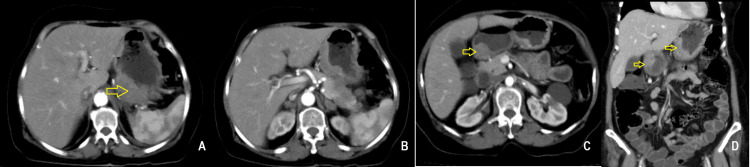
Contrast-enhanced computed tomography images in axial sections showing asymmetrical, heterogeneously enhancing wall thickening involving the proximal stomach along the lesser curvature (A), with focal infiltration of the pancreas (B), asymmetric thickening in the pylorus and duodenal bulb (C), and a coronal section showing involvement of both the lesser curvature and pyloroduodenum (D).
